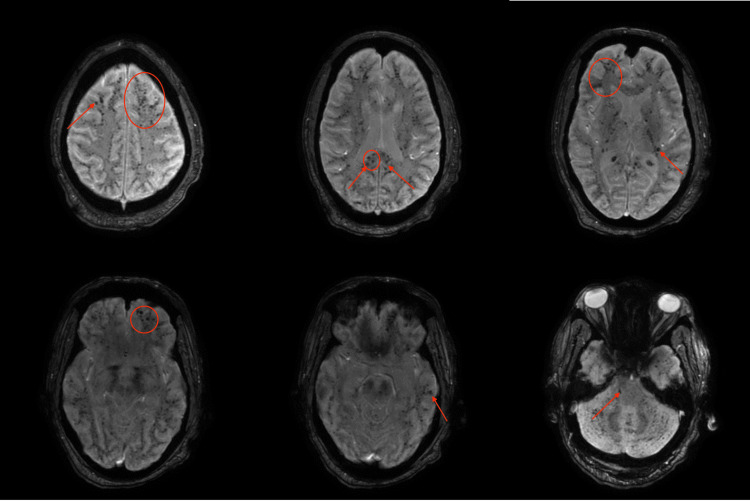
Axial GRE sequence of MRI brain that is showing diffuse multiple hemorrhages related to FES in our patient (some lesions outlined by arrows and circles)
